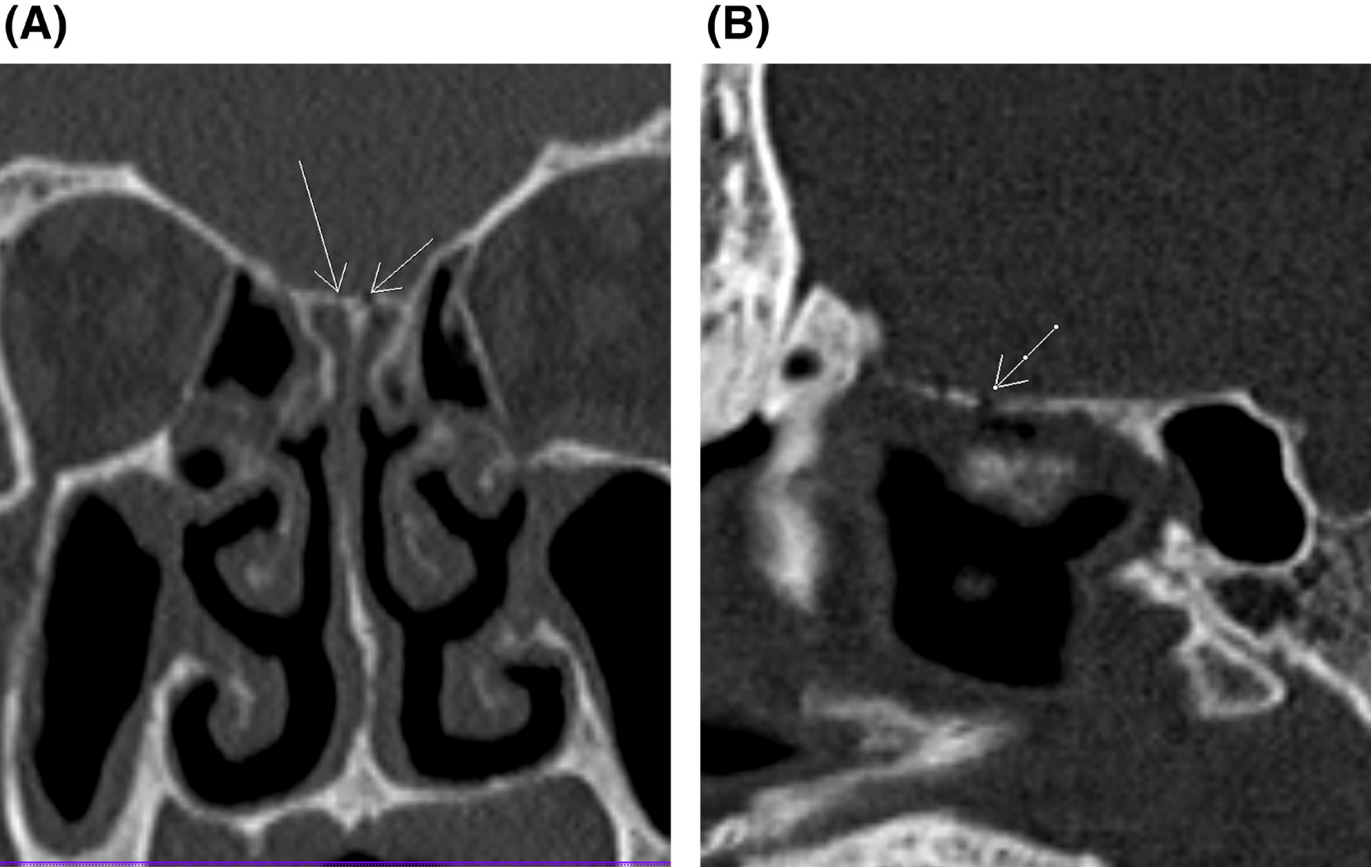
CT scan of the head with bone window. (A) Linear undisplaced fracture of cribriform plate and right lamella in coronal plane (arrows). (B) Fracture of cribriform plate in sagittal plane (arrow).
